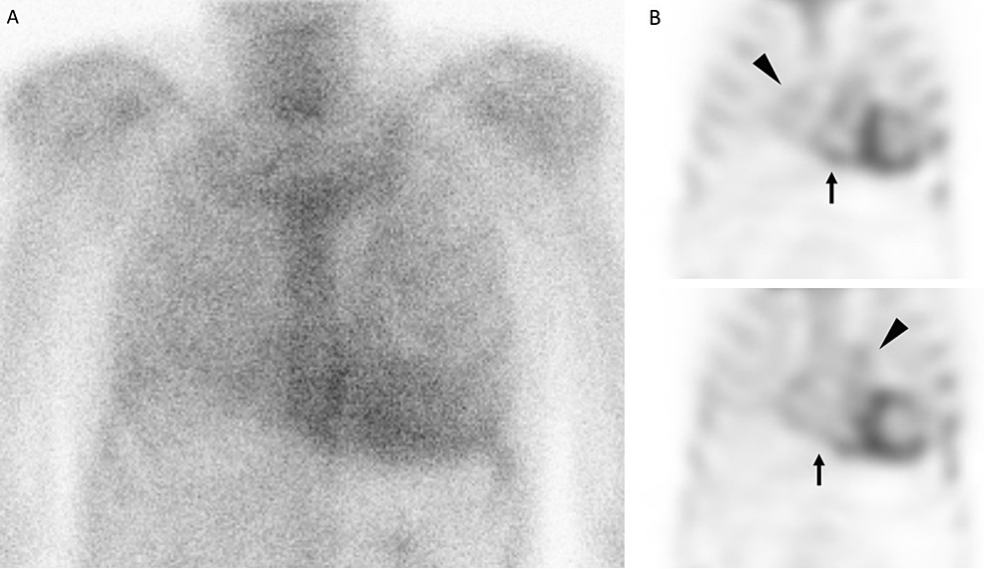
The patient's scintigraphy images Myocardial imaging with technetium-99m-pyrophosphate demonstrates more tracer uptake of the heart than the bones (A). Coronal images show tracer uptake not only in the left ventricle but also in the right ventricle (B, arrows), along with biatrial uptake (B, arrowheads).
